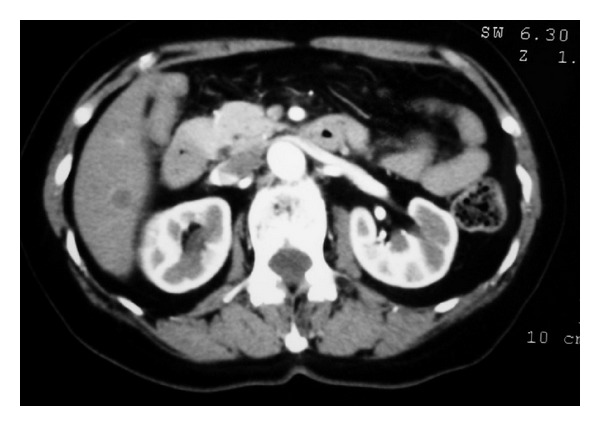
Computed tomography (CT) with contrast demonstrated a 11 mm low-density lesion with mild ring enhancement on the rim in the arterial phase, located in the liver segment VI.
